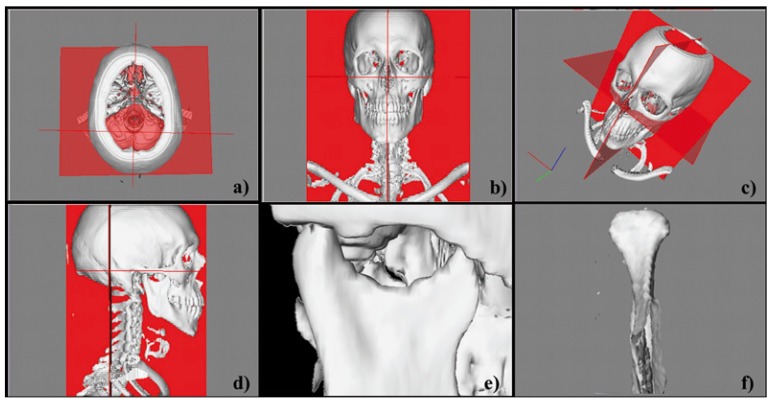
Isolation of 3D-CT reconstruction model.
